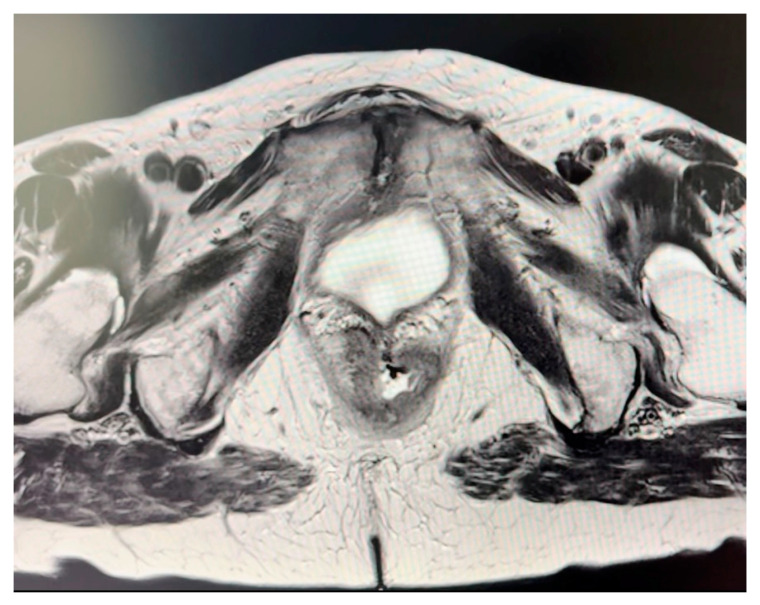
Magnetic resonance imaging (MRI) of the anal canal showing fistula formation into the vagina.
